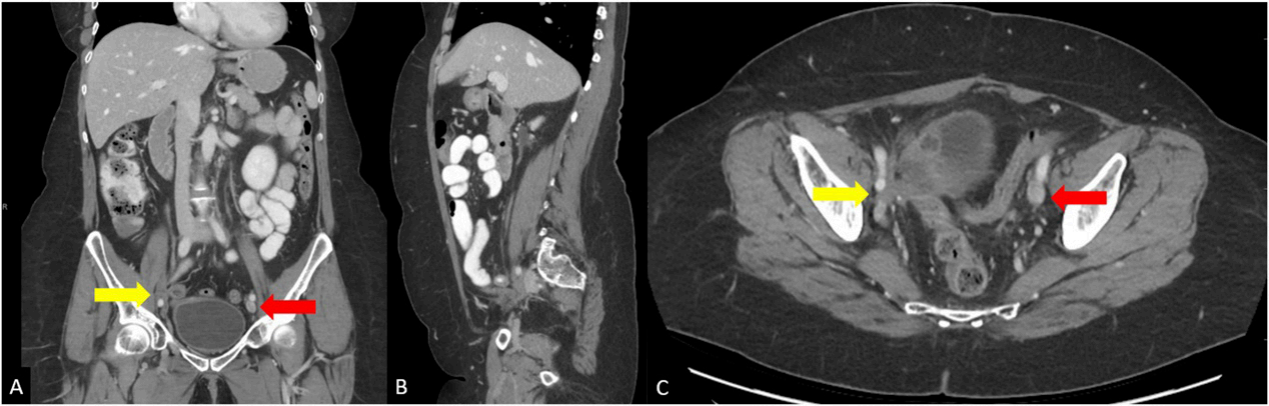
(A) Coronal, (B) sagittal, and (C) axial CT occlusion of the right external iliac vein with nearby soft tissue pelvic mass (yellow arrow). Normal opacification of the left external iliac vein can be seen (red arrow).
